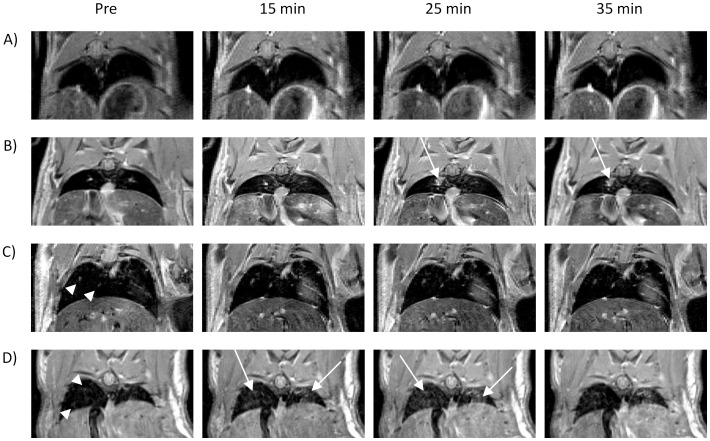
MR images over the 35 minute eqMRI time course. A) control lungs, B) a discrete gadolinium enhancing tumor nodule (25 minutes) (low tumor cell seeding model), C) tumor nodules visible on the pre contrast scan (low tumor cell seeding model), D) tumor presentation representative of a high tumor cell seeding model lung at day 14. Tumors visible on pre contrast (white arrowheads) and tumors only visible with gadolinium enhancement (white arrows) are indicated.
